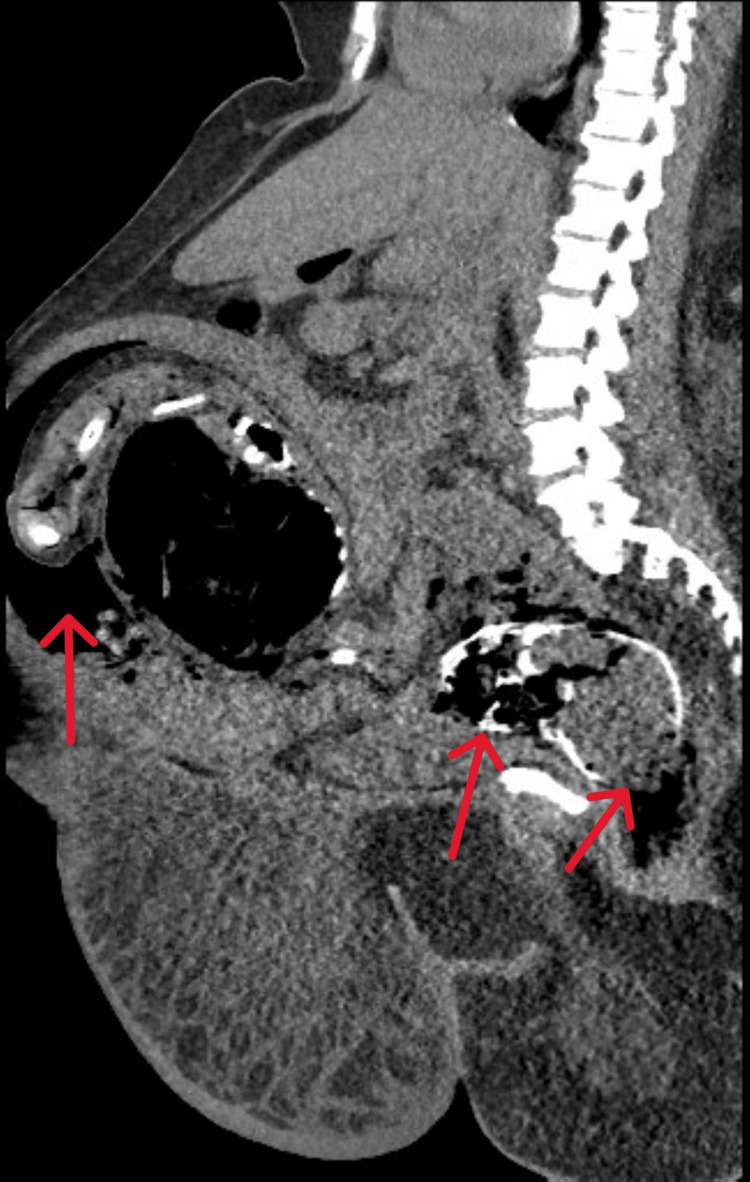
CT of the abdomen and pelvis without contrast sagittal view. Gravid uterus with the fetus measuring up to 36 cm from the calvarium to the lower pelvis. There is air in the abdominal cavity, the umbilical cord, and placental vessels (left arrow). There are extensive gas bubbles in the subdural space of the skull, thoracic cavity, abdominal cavity, and anterior soft tissue of the head and neck (middle arrow). There is an acute fracture of the fetal calvarium and there are adjacent large locules of air in the maternal vaginal canal (right arrow). The fetal bowel is dilated.
